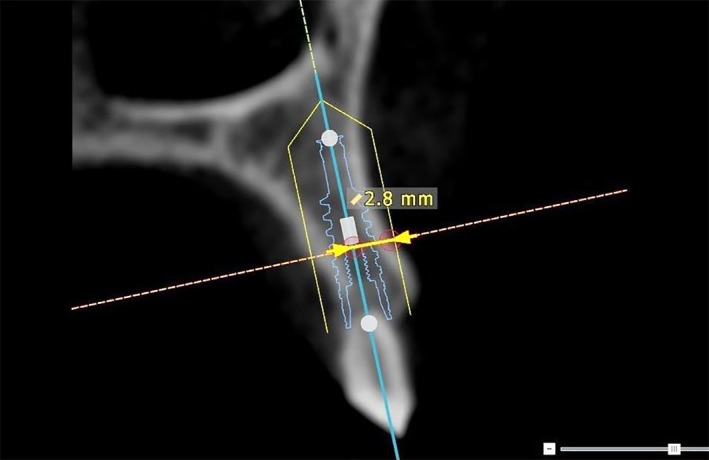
Measurement on a pretreatment cone‐beam computed tomography from the central axis of a planning implant, placed in the position where the actual implant will be after surgery, to the outer surface of the buccal bony wall of a failing natural tooth with the planning program NobelClinician
